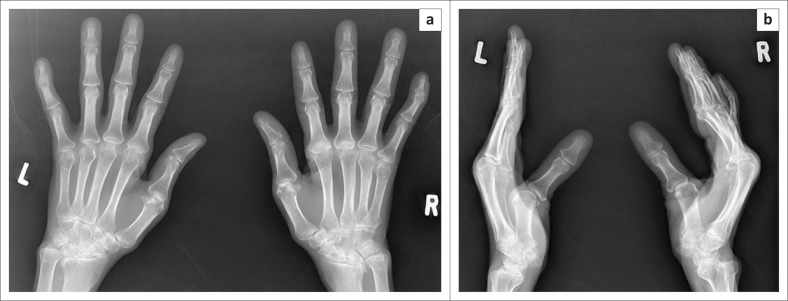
(a) Frontal and (b) lateral radiographs of both hands of an HIV-positive patient presenting clinically with reducible deformities and arthralgia. Rheumatoid factor and anti-nuclear factor were negative. Lateral radiographs show subluxations at the metacarpophalangeal joints bilaterally as well as the distal interphalangeal joints of both 5th fingers. The right hand is more affected than the left, with the left hand demonstrating more reducible deformities on the frontal radiograph compared to the right hand.
